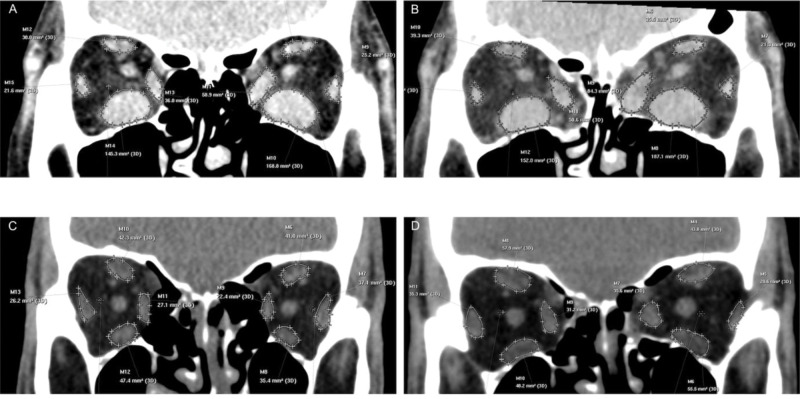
Computed tomography measurements of the recti muscle areas. A and B. Preoperative (A) and postoperative (B) measurements of a patient submitted to inferomedial wall orbital decompression (IM-OD). C and D. Preoperative (C) and postoperative (D) measurements of a patient submitted to balanced medial plus lateral wall orbital decompression (ML-OD).
